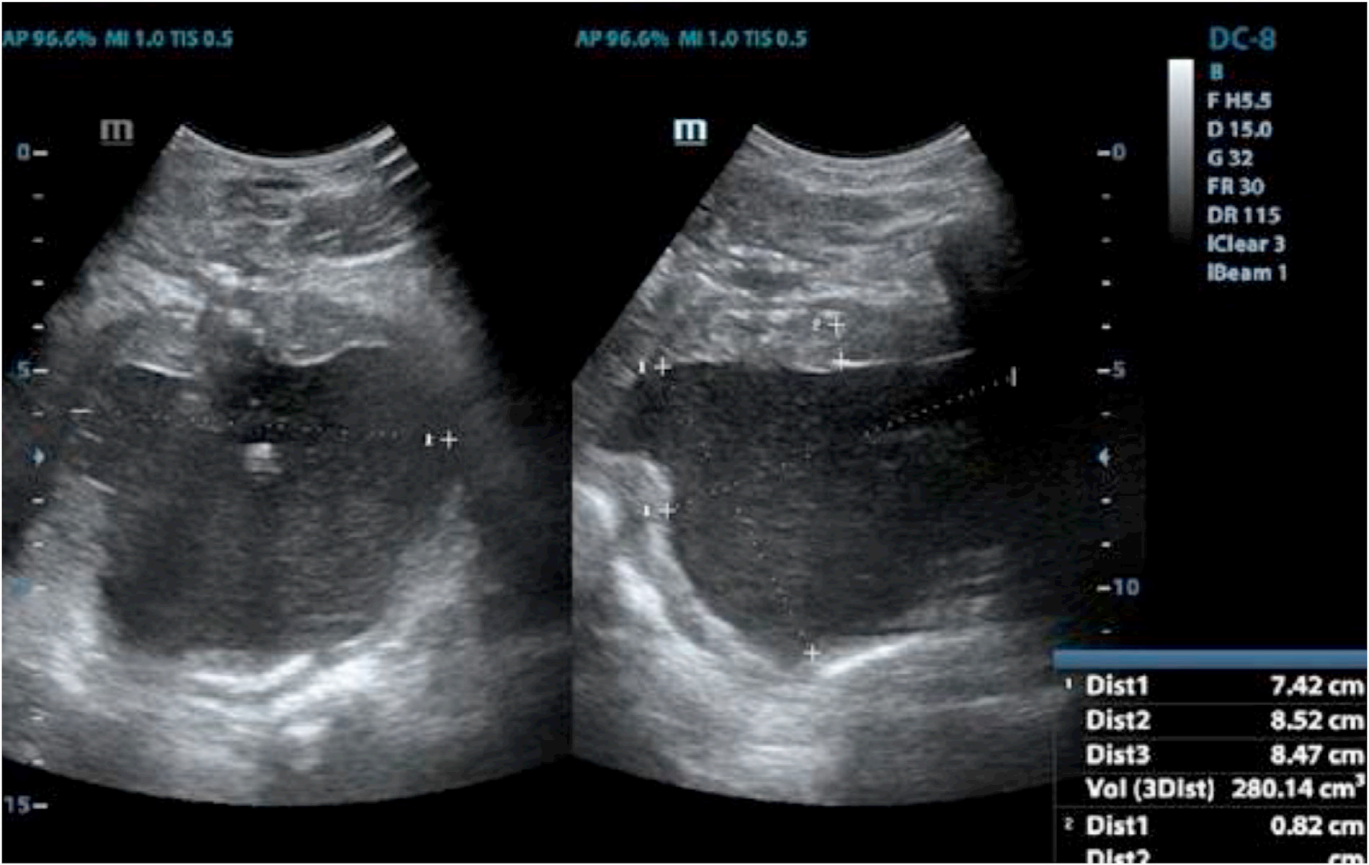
Postsurgical bladder ultrasound. Urinary bladder: Presents a diffuse wall thickening (up to 14 mm). Presence of 2 diverticula.
